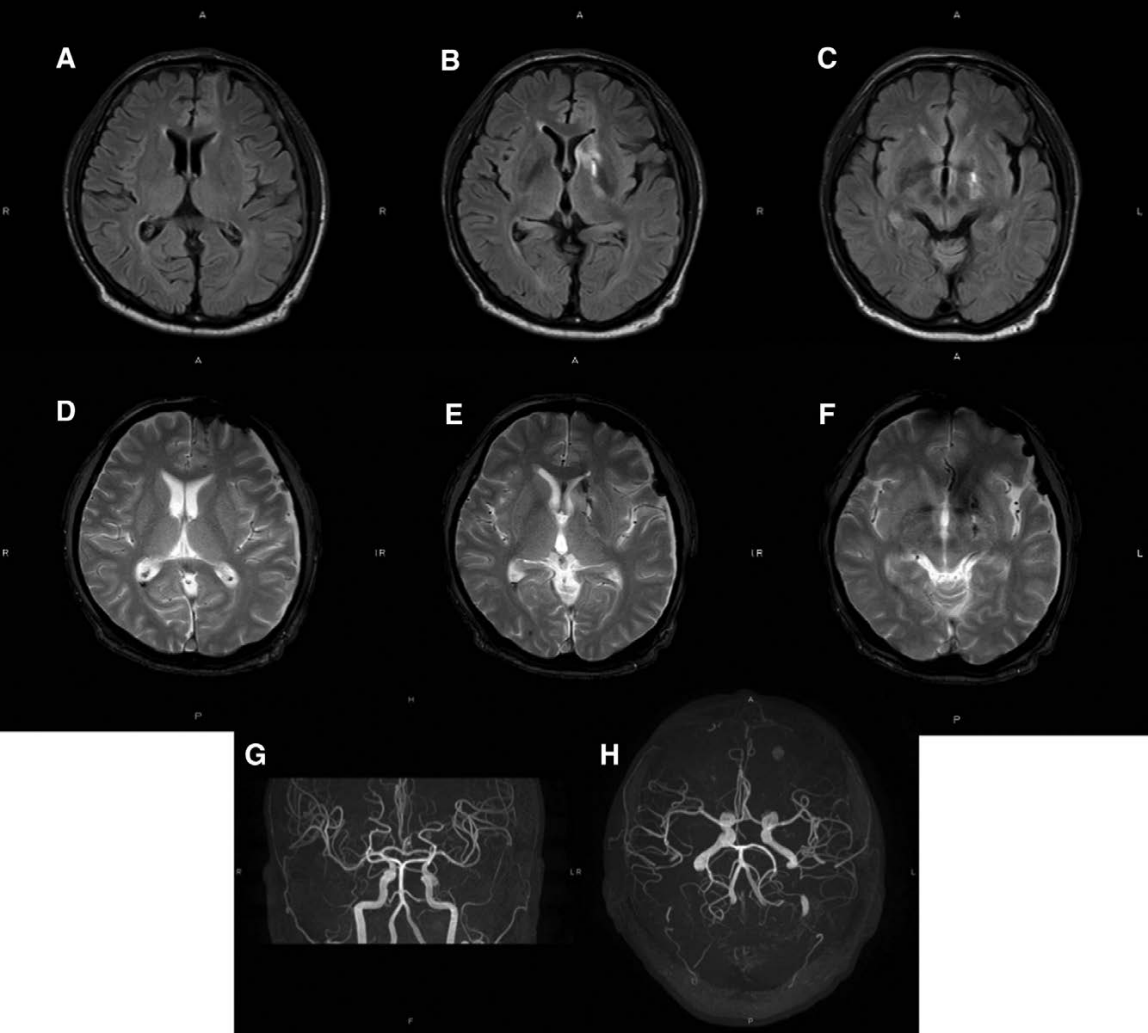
MR images of the brain. Axial FLAIR images acquired on hospital day 5 show no brain abscess or hemorrhagic enlargement (A–C). On axial T2* imaging, a small amount of hemorrhage is observed along the route taken by the gimlet (D–F). There is no evidence of traumatic cerebral aneurysm formation on magnetic resonance angiography (G and H).
